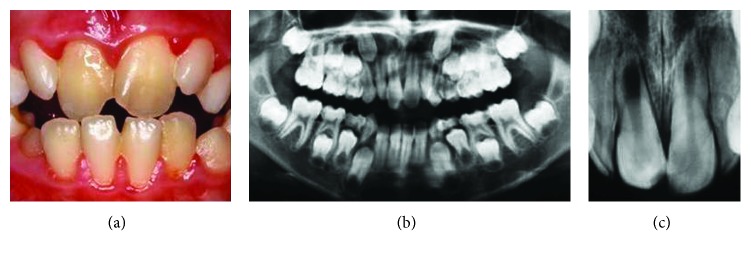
(a) Intraoral view of central incisors. (b) Panoramic radiograph. (c) Periapical radiograph before treatment.
